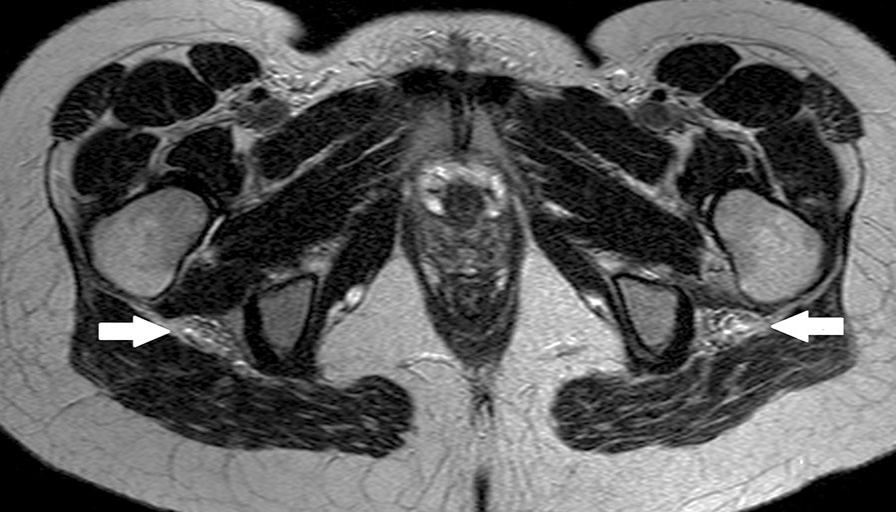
Axial T2-weighted MRI of the pelvis. It shows bilateral enlarged sciatic nerves (*arrows*) with increased signal intensity. Note that single fascicles are separated from each other but the fascicular structure of the nerve is preserved
